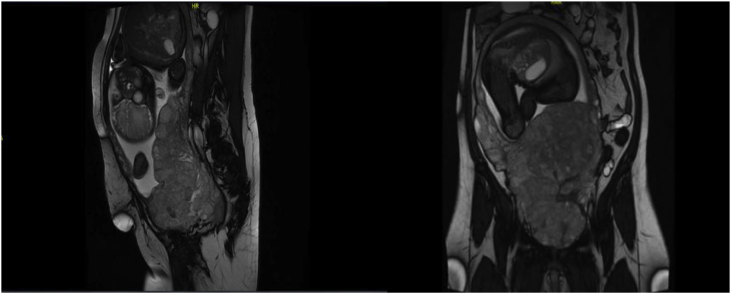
Magnetic resonance imaging showing placenta percreta with invasion of the urinary bladder fundus. There was also suspicion of invasion to the anterior wall of the rectum and lower sigmoid colon associated with congested pelvic vessels.
